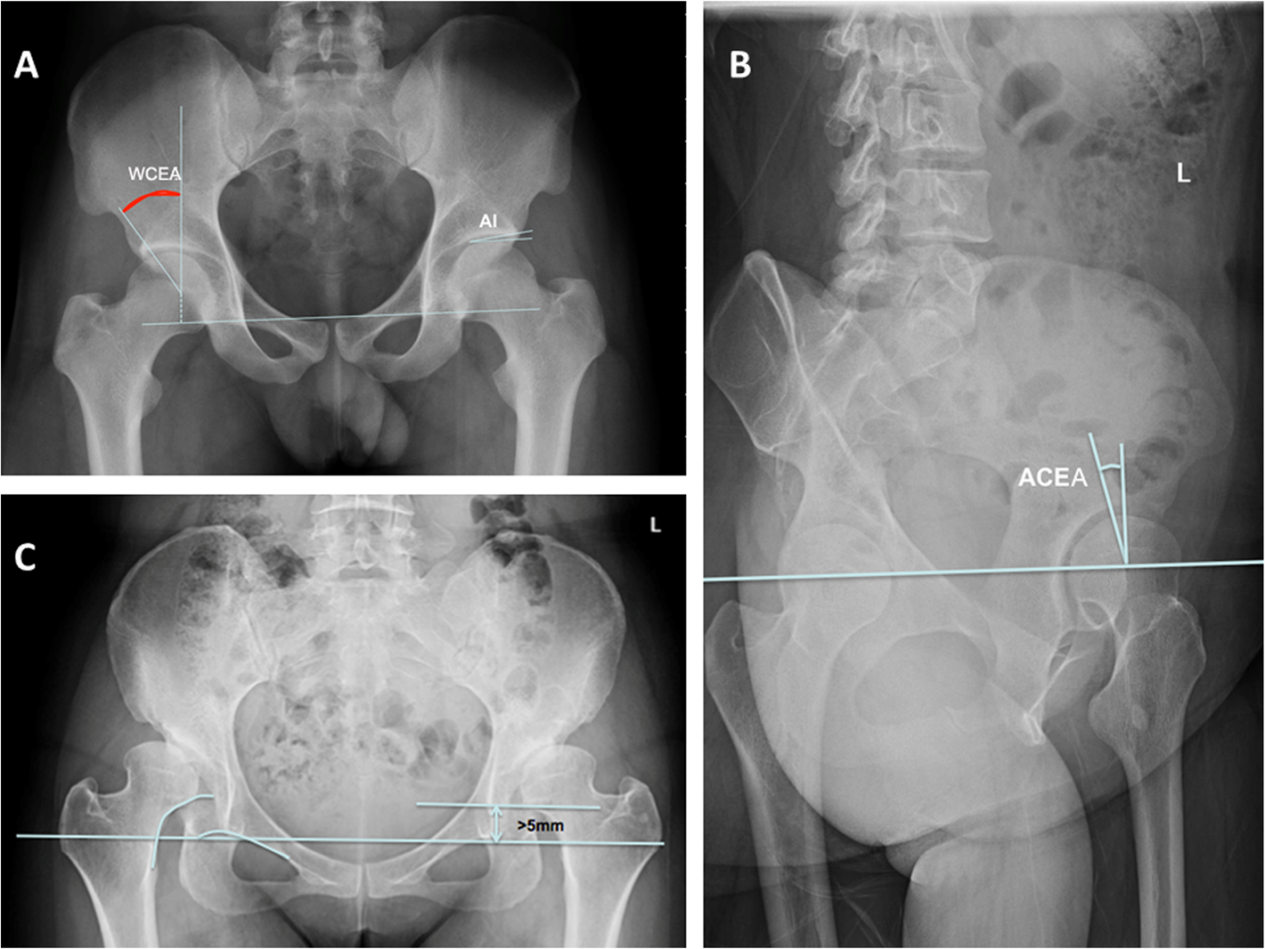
Measurements of radiographic parameters in anteroposterior and 65° false⁃profile pelvic X-ray radiographs in the standing position. a Lateral center-edge angle (LCEA) is the intersection angle between the vertical center line of the femur and the lateral edge of the acetabulum; AI is the intersection angle formed with the connection line between the acetabular endocoxa and acetabular outer margin and the pelvic horizontal line. b Anterior central edge angle (ACEA) measurement on 65° obliquity on the pelvis of X-ray image. ACEA is the intersection angle formed with the vertical center line of the bilateral femoral head and the anterior margin of the acetabulum. c Discontinuity of Shenton line at the pelvis AP position on the X-ray image is defined as a more than 5 mm distance away from the connection line of the obturator superior border vertex and the head and neck junction of the femoral neck
